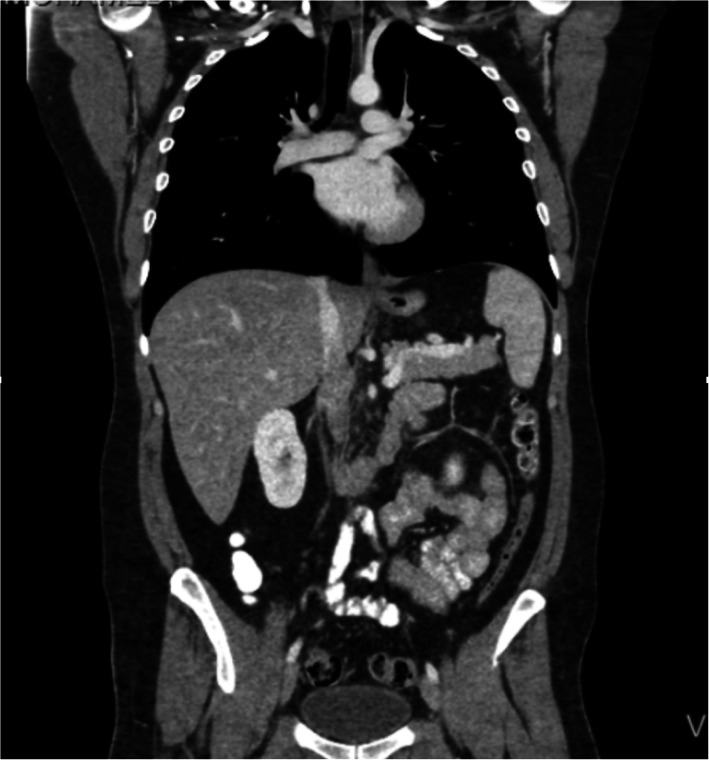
CT of the chest, abdomen, and pelvis performed in the postoperative setting, showing no evidence of a leak or significant collection
